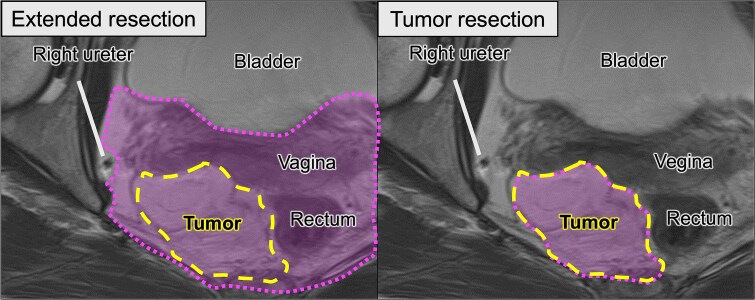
Comparison of surgical options based on preoperative MRI. Left (extended resection): The hypothetical resection area (dotted line) includes the tumor (dashed line), rectum, and vagina, corresponding to the multivisceral resection approach. Right (tumor resection): Planned organ-preserving resection where the tumor is delineated in yellow, and the surrounding organs are preserved.
